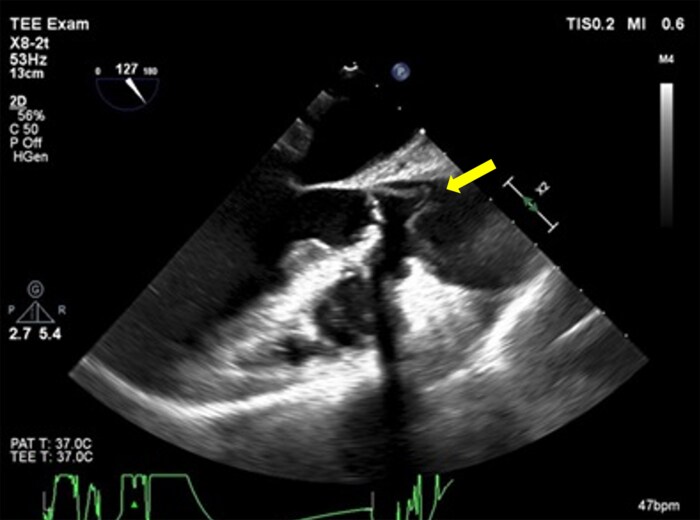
Intraoperative transoesophageal echocardiography in Case 1 (mid-oesophageal aortic valve long-axis view) demonstrates an intimal flap (arrow) and false lumen in the ascending aorta.
